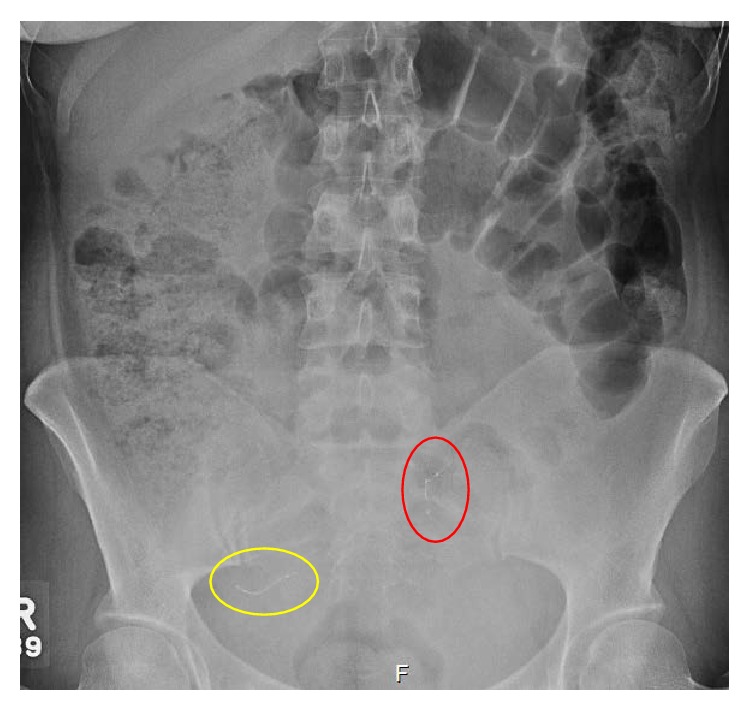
Patient Number 1, postpartum abdominal X-ray 8/30/2014: Essure (circled in red) in the abdomen.
